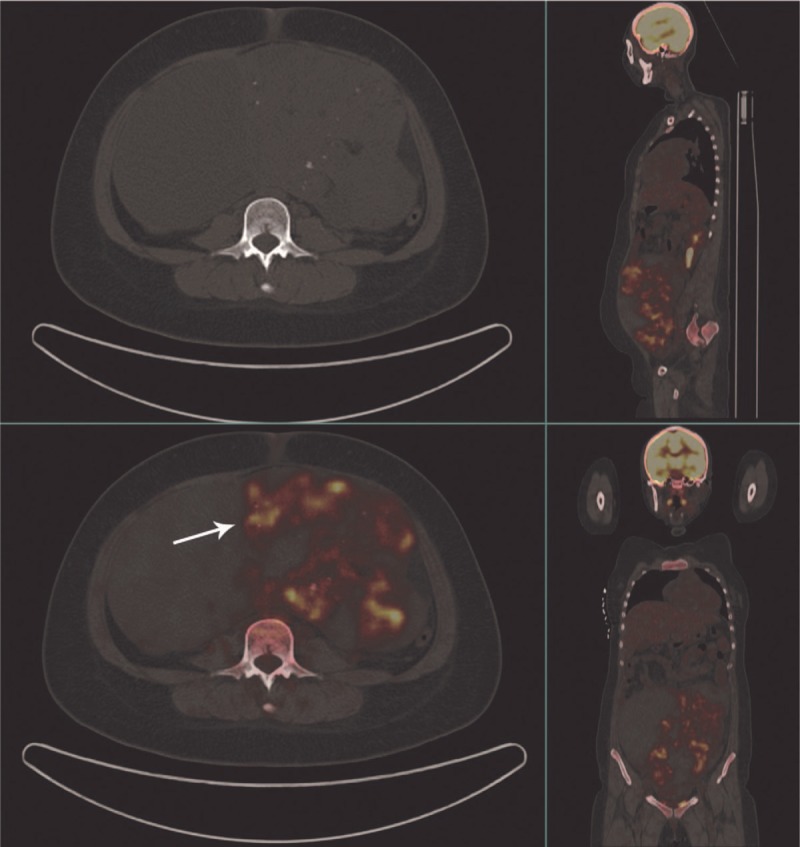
Before surgery, CT images demonstrated a large mass with a maximum diameter of 25 cm with solid, cystic, fat, and calcified components. 18F-FDG PET/CT showed pathological FDG uptake in solid components of the abdominopelvic mass. Intensely increased FDG uptake was also seen in the retroperitoneal lymph nodes of the bilateral pelvic wall and bilateral iliac fossa. CT = computed tomography, 18F-FDG PET/CT = fluorine-18 fluorodeoxyglucose positron emission tomography/computed tomography.
